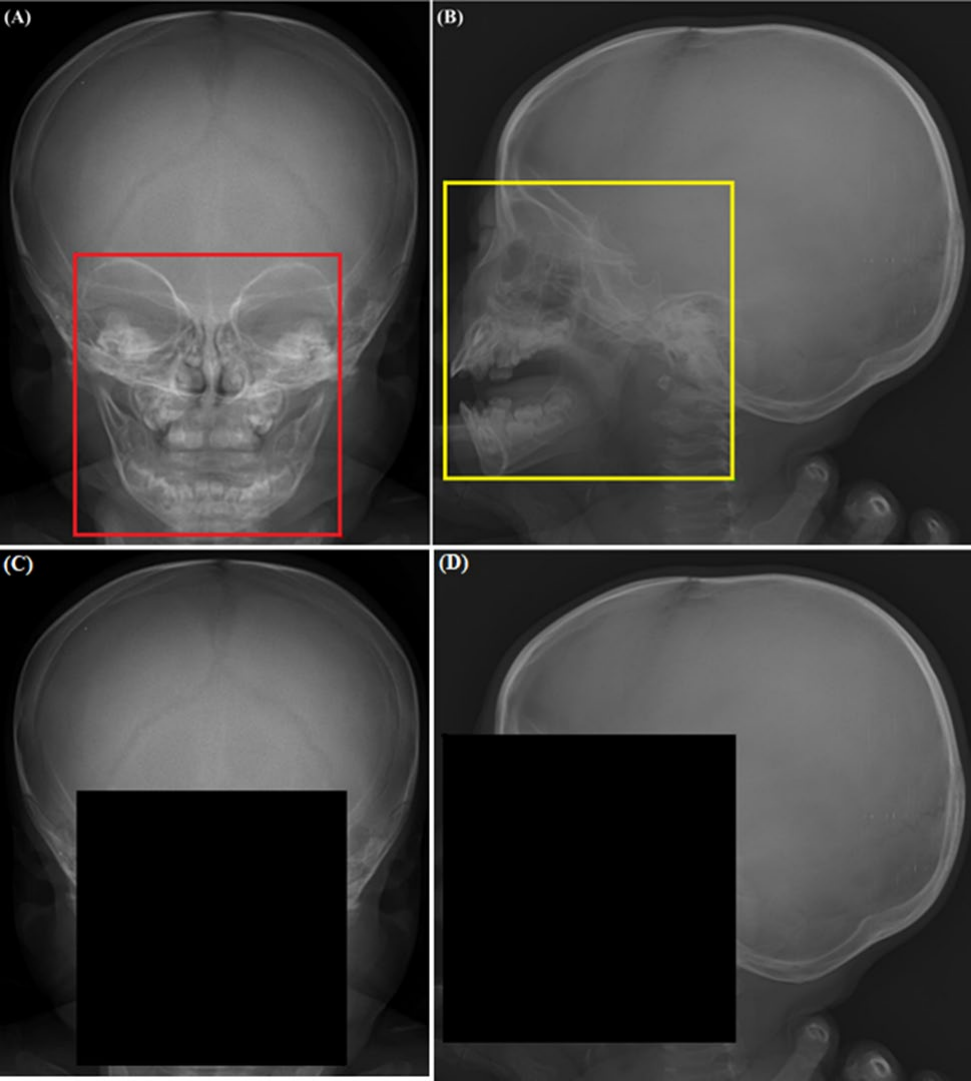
The defined region of exclusion (ROE) in the skull X-ray for image tailoring. (A) Anteroposterior (AP) or Town’s view skull X-ray showing the defined ROE. The borders of the ROE extend from the upper margin of the supraorbital rim to the lower margin of the mandible. (B) Lateral skull X-ray with the ROE including the supraorbital rim, the foremost part of the mandible, and the posterior margin of the cervical spinous process. (C) Post-processed AP or Town’s view skull X-ray. The region below the upper margin of the ROE has been removed. (D) Post-processed lateral skull X-ray. A square box, defined by the upper and right margins of the ROE, has been removed.
